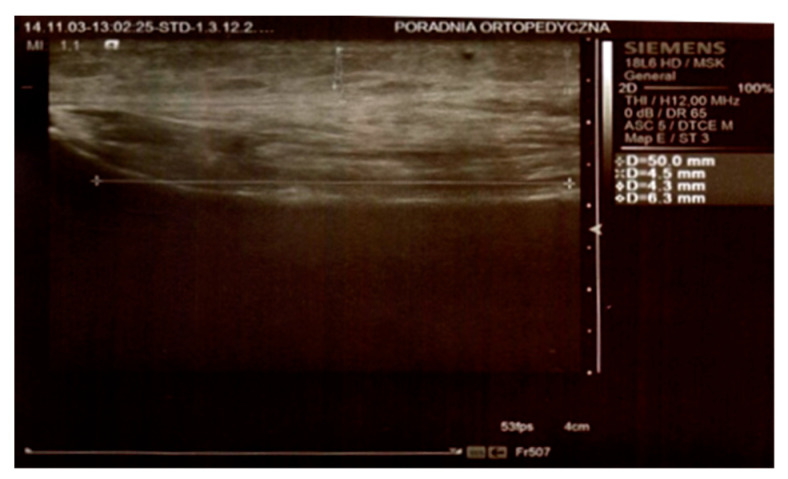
Ultrasound scan. Measurements 25 mm below the neck of the fibula and 50 mm below the neck of the fibula.
